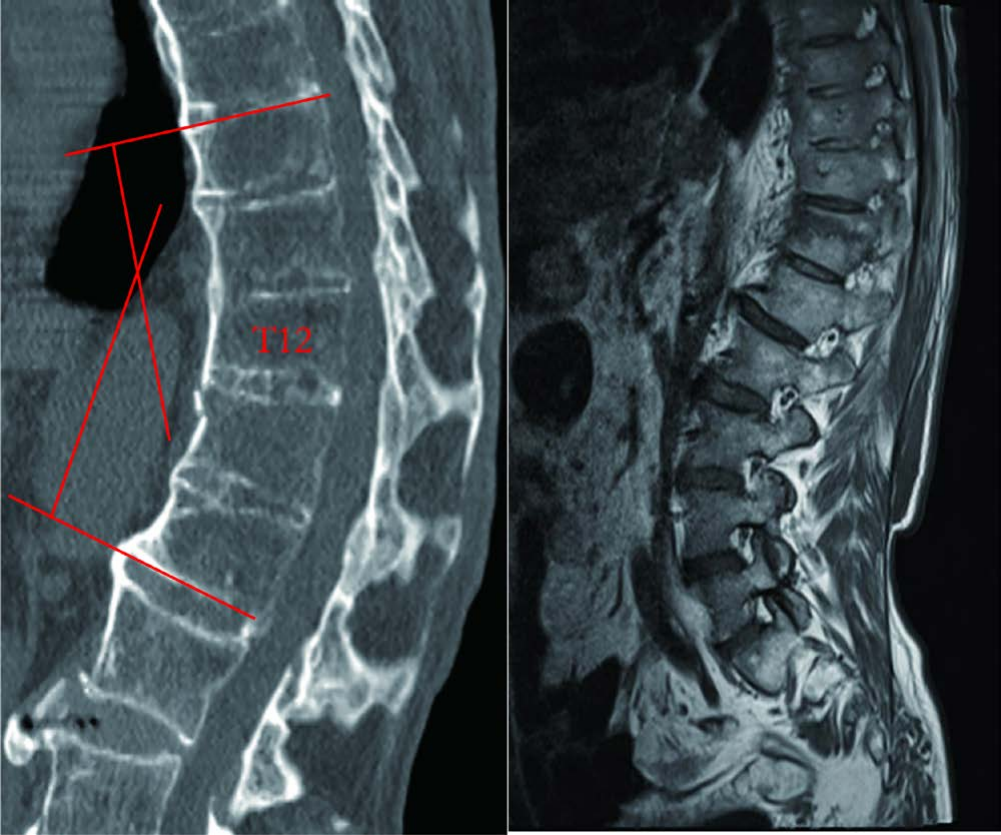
A 61-year-old patient AS with T12 vertebral compression fracture was treated with O-arm navigation assisted by wiltse approach internal fixation combined osteotomy. The pre-operative kyphosis Cobb angle is 38.6 degrees. CT indicated “bamboo” changes in the spine (on the left). MRI indicated T12 vertebral compression fracture (on the right left).
